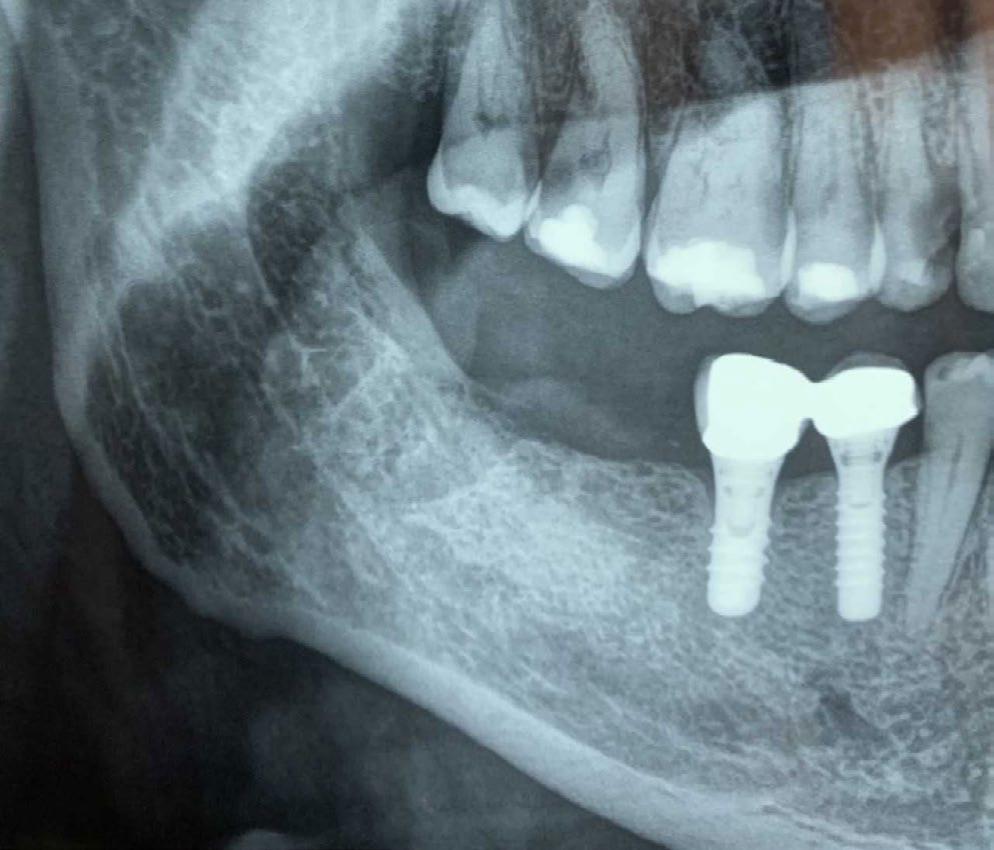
Orthopantomography (OPT) exhibited from the patient during the first visit, revealing the nonhealing alveolus 4 mo after tooth extraction
